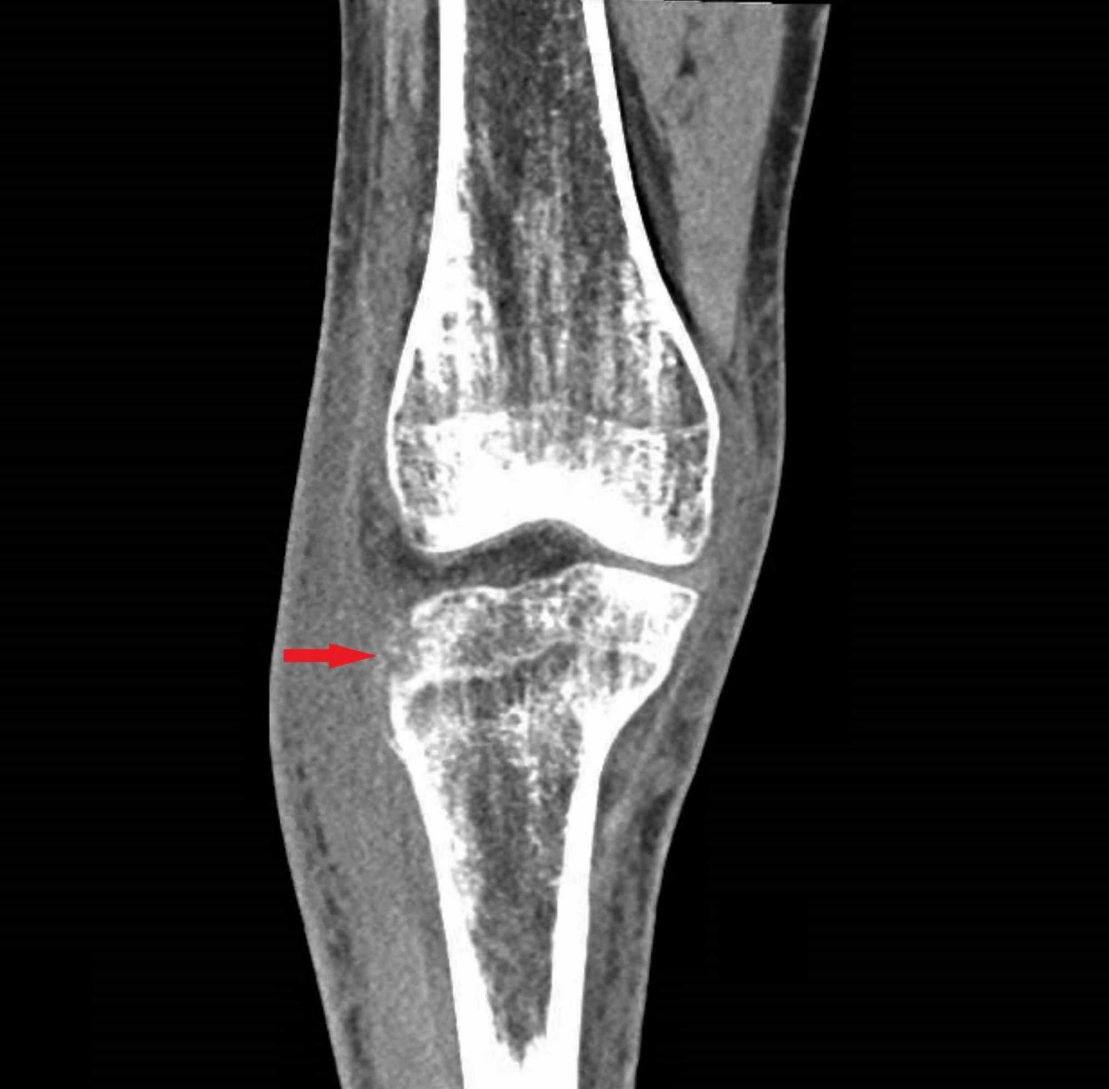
Computed tomography (CT) scan of the right lower extremity showing cortical irregularity (red arrow) around the proximal tibial metaphysis
